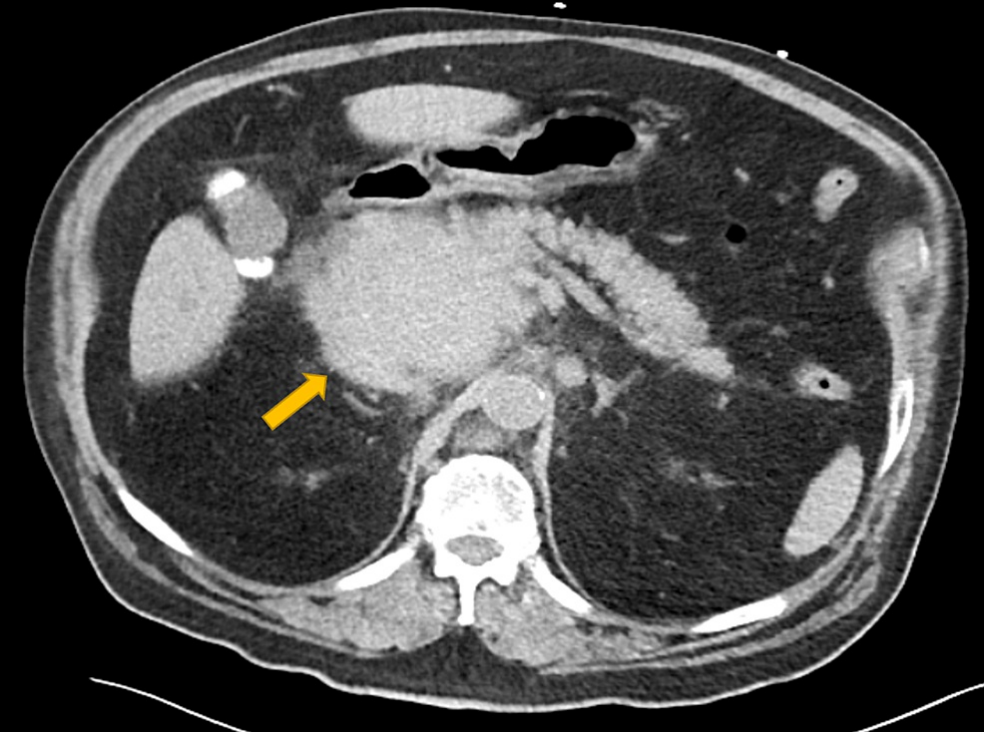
CT abdomen and pelvis without contrast demonstrating large round mass (8.5 x 7.8 cm) within the head of the pancreas (orange arrow) CT: computed tomography
